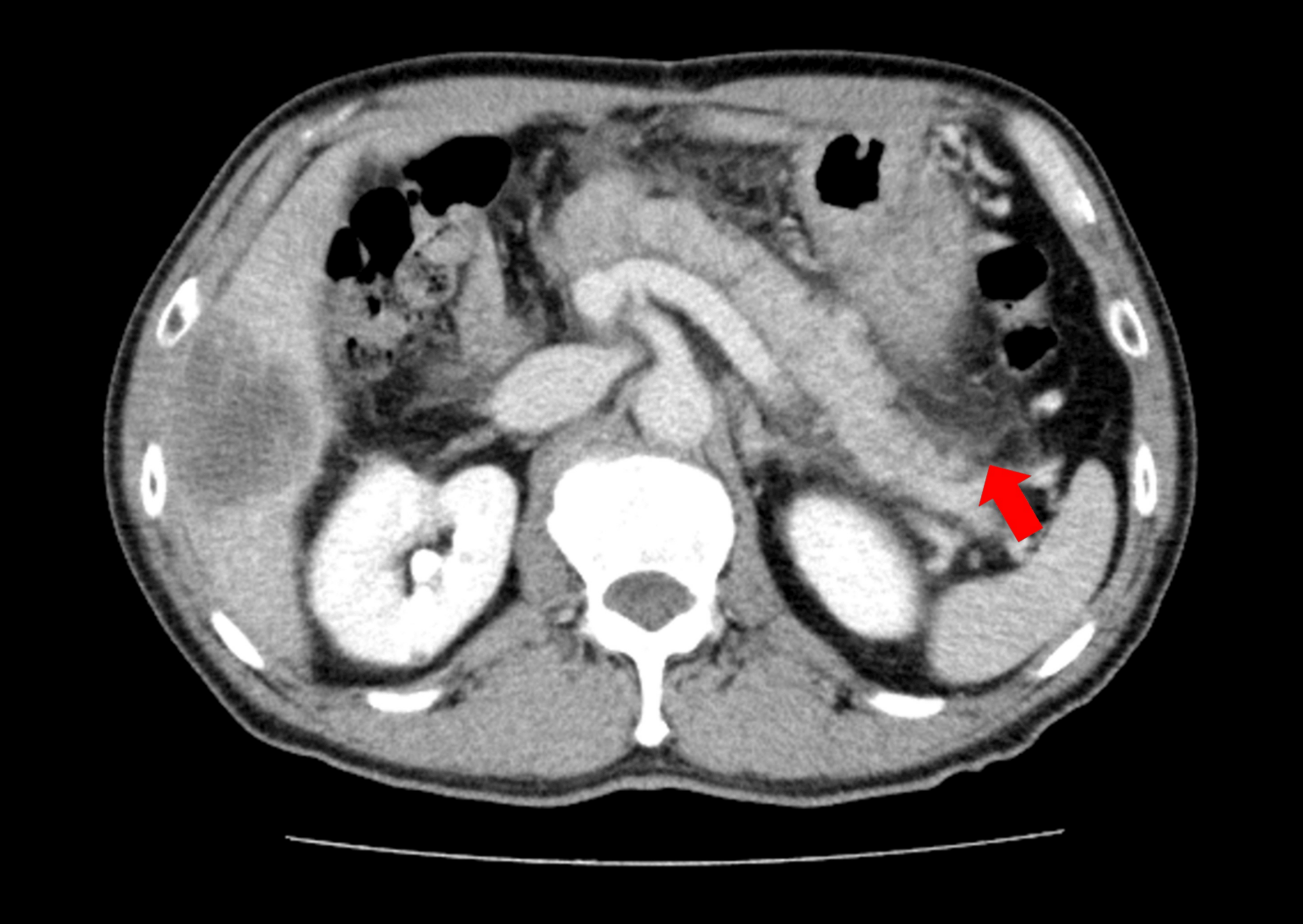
Follow-up CT of the remnant pancreas (April 2024) Contrast-enhanced abdominal CT taken in April 2024 shows persistent but stable inflammatory changes around the remnant pancreas (red arrow), with no new fluid collection or necrosis.
